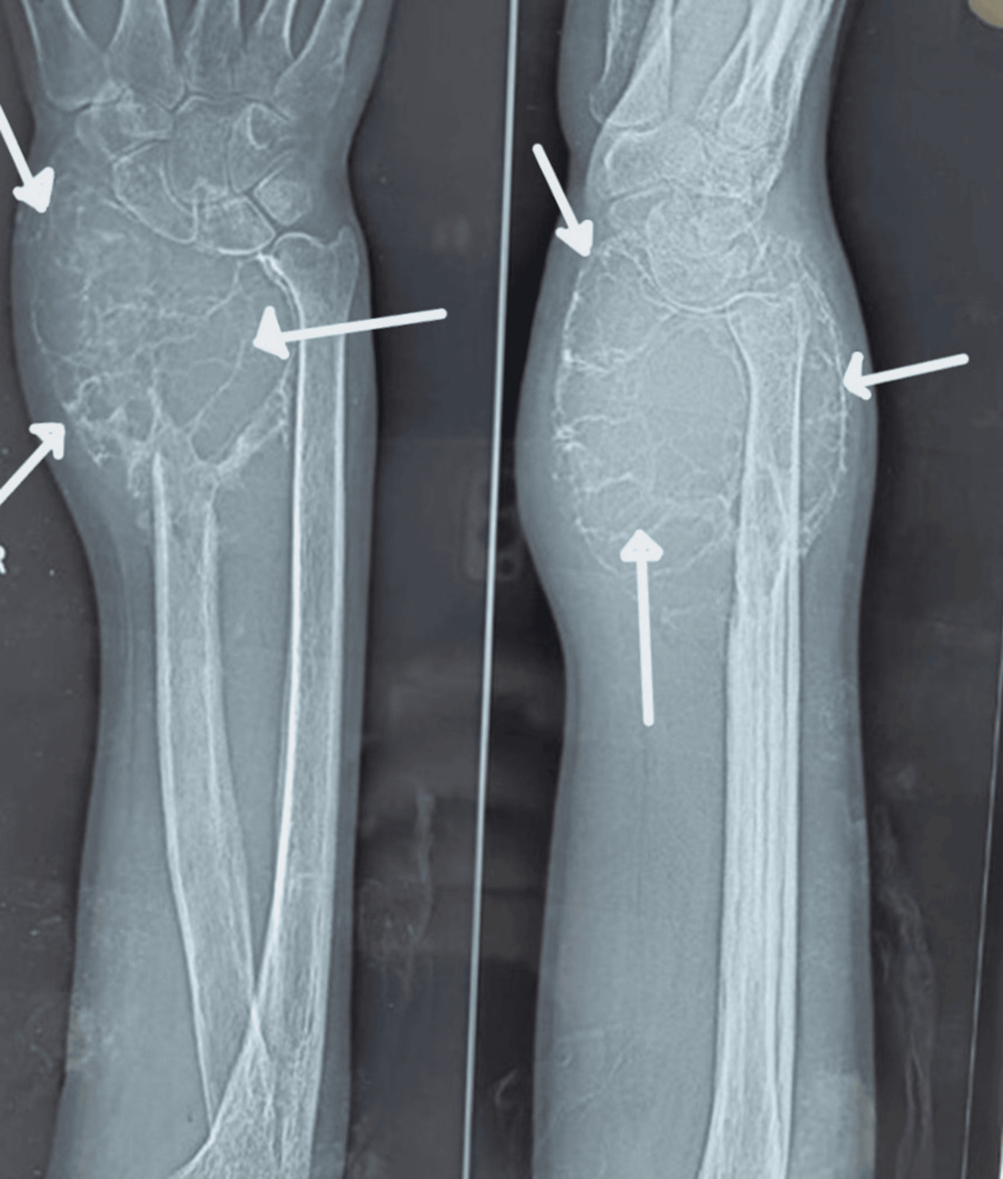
Pre-operative radiograph Shows an expansile, lobulated, lytic lesion involving the distal radius (white arrows) with cortical destruction Campanacci Grade III Giant cell tumor (GCT) in a 31-year-old patient.
